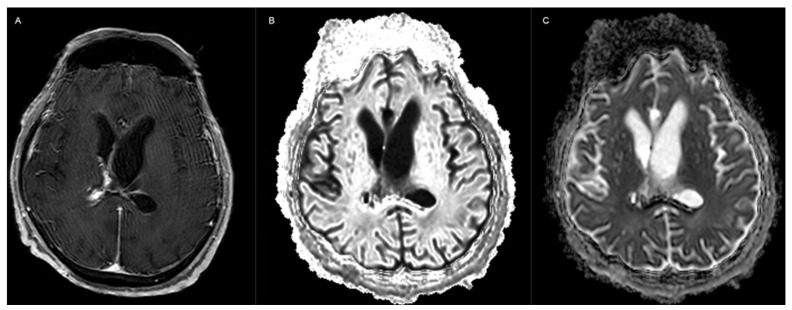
Magnetic resonance imaging scan (A) of the patient revealed contrast enhancement of the walls of the enlarged ventricular system (B) with restricted diffusion, consistent with ventriculitis (C).
